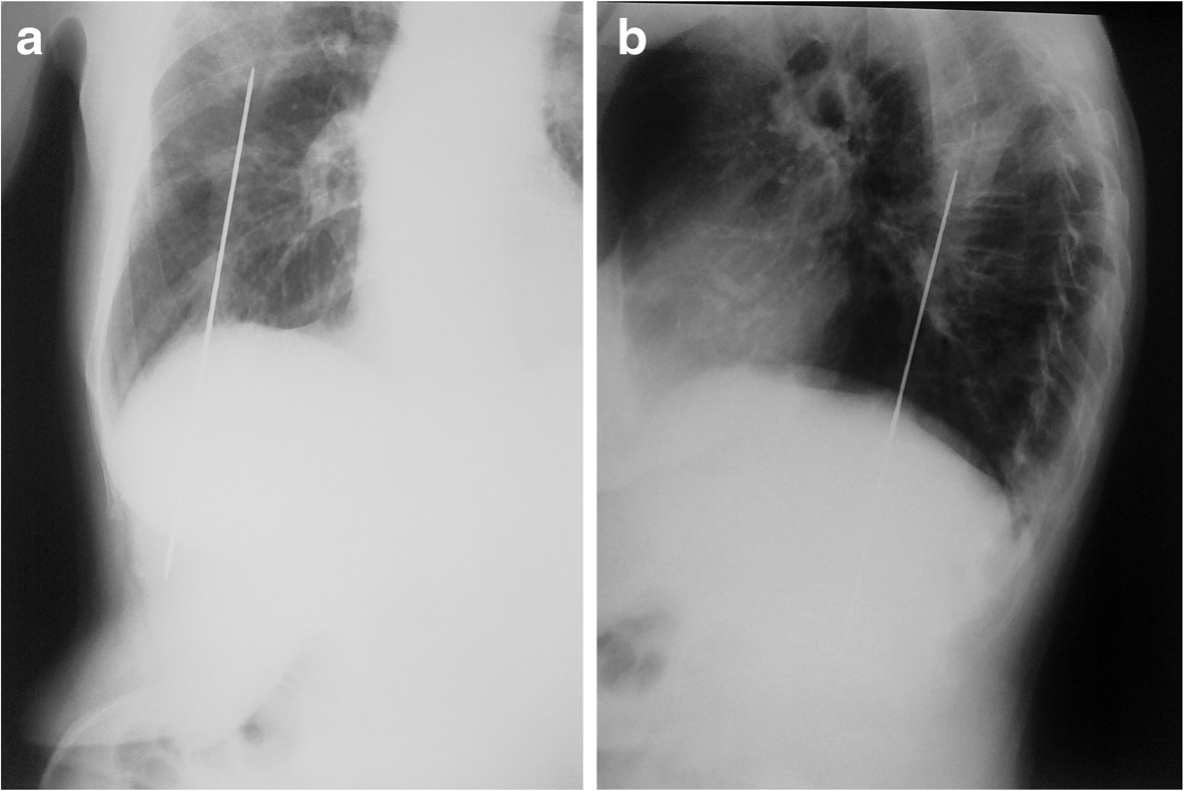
a and b Follow-up X-rays (PA and lateral) focused on the foreign body demonstrating its location as thoraco-abdominal
